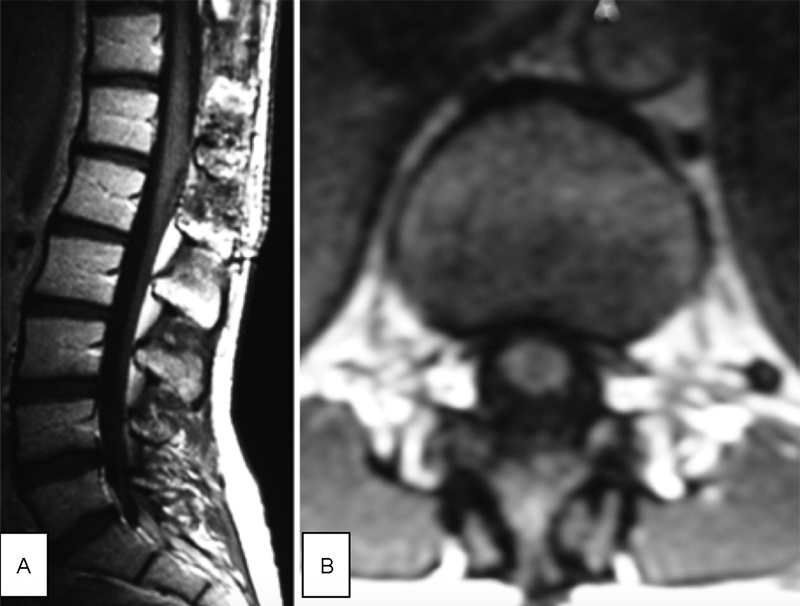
Postoperative control MR imaging performed 6 months after operation showed no evidence of the tumor. (
